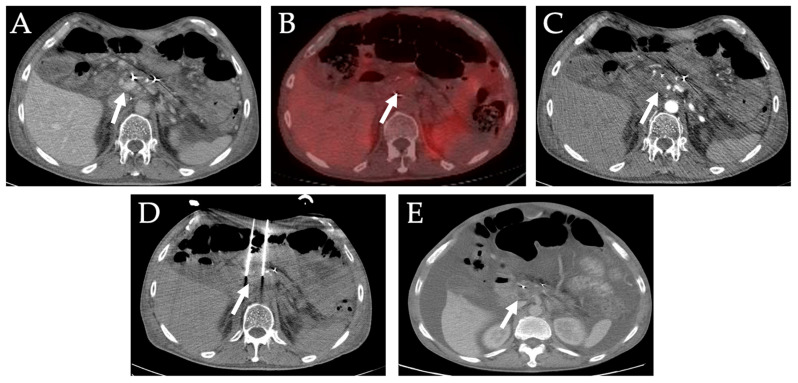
Irreversible electroporation of locally advanced pancreatic mass. A 57-year-old male with a history of pancreatic cancer presented with nonspecific epigastric pain. He was found to have locally advanced pancreatic head tumor (white arrow). He underwent chemotherapy and radiation and follow up CT and PET images (A–C) showed persistent locally advanced pancreatic head tumor. (D) He subsequently underwent IRE. (E). One-month follow up CT scan shows no residual disease.
